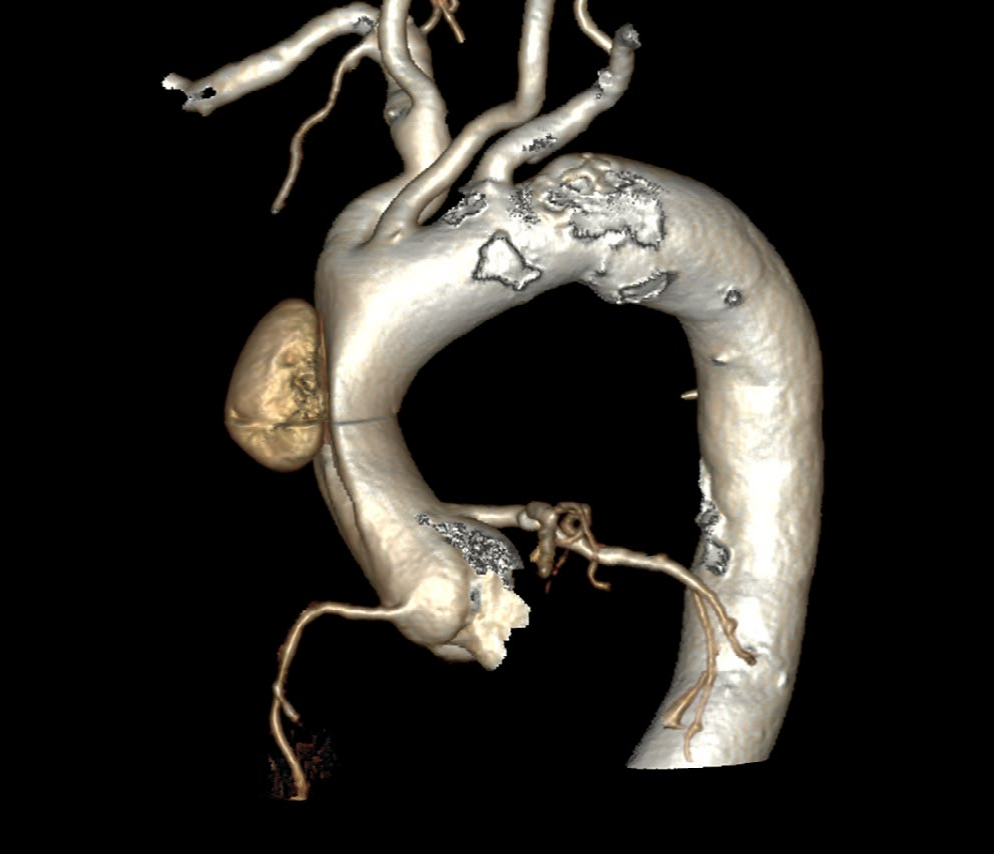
Computed tomography 3D‐reconstruction of the aortic arch displaying Stanford type A/DeBakey type II acute aortic dissection.
